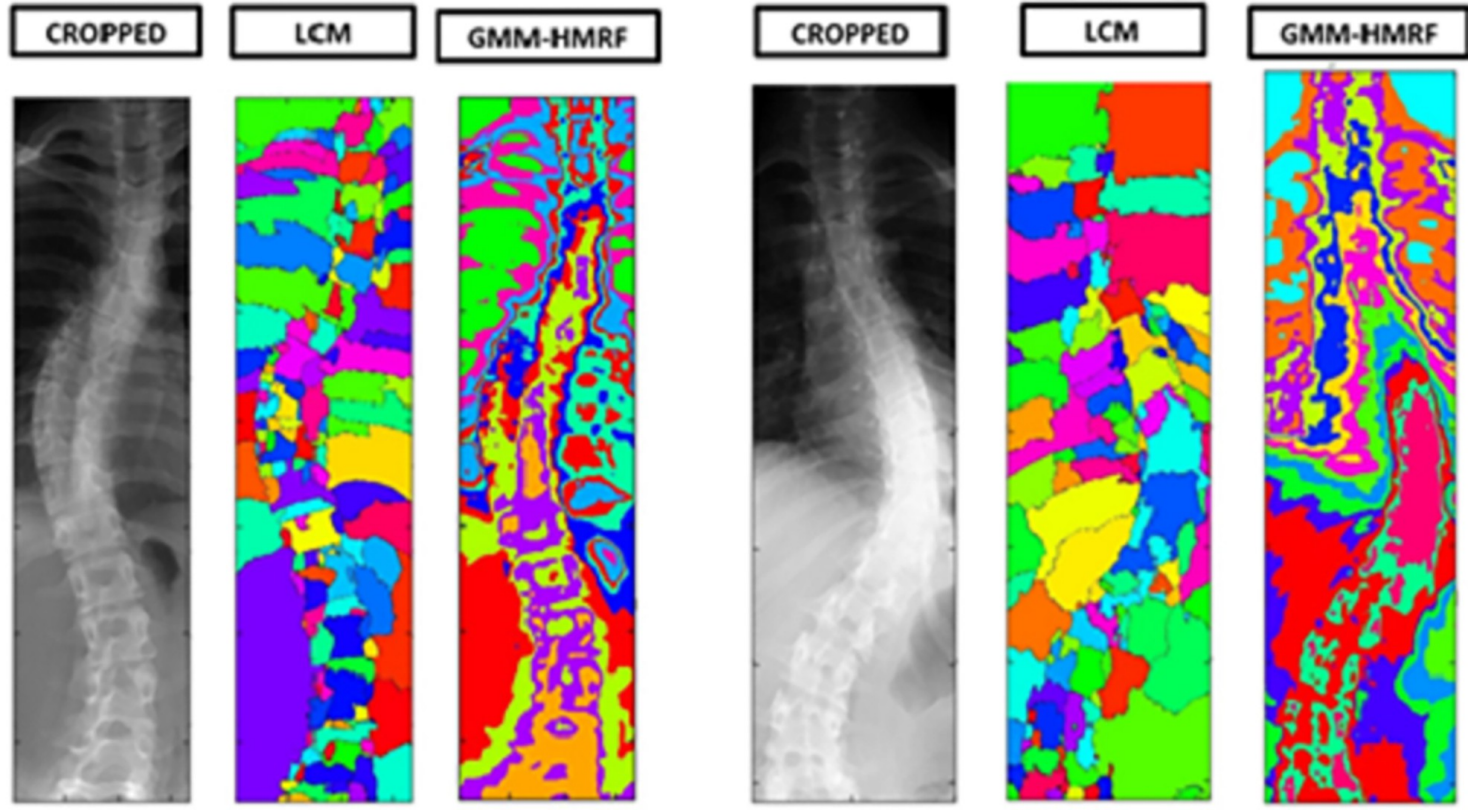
X-ray Image Segmentation of Scoliosis in (a) Thoracic region (b) Thoraco-Lumbar region.
